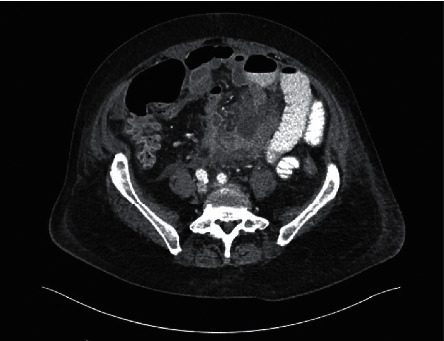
Repeat computed tomography (CT) of the abdomen and pelvis with new peritoneal enhancement suggestive of peritonitis.
